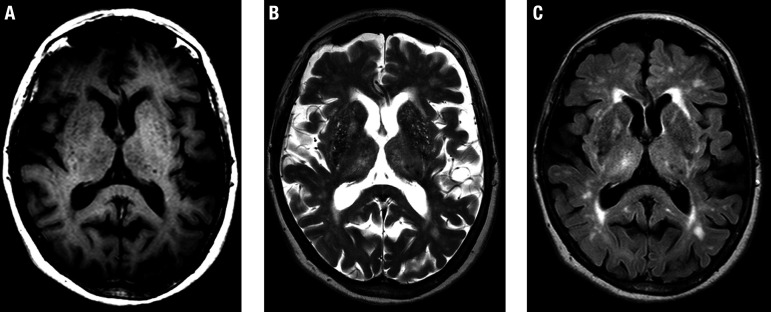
Prominent perivascular spaces. A 75-year-old woman with cognitive decline. Axial T1-weighted (A), T2-weighted (B) and FLAIR (C) images demonstrate multiple prominent perivascular spaces in bilateral basal ganglia regions, smaller than lacunes (see Figure 2), but also with signal intensity similar to CSF in all sequences. There is no T2/FLAIR hyperintense rim. Note the concomitance of white matter hyperintensities of presumed vascular origin on T2-weighted (B) and FLAIR (C) images.
